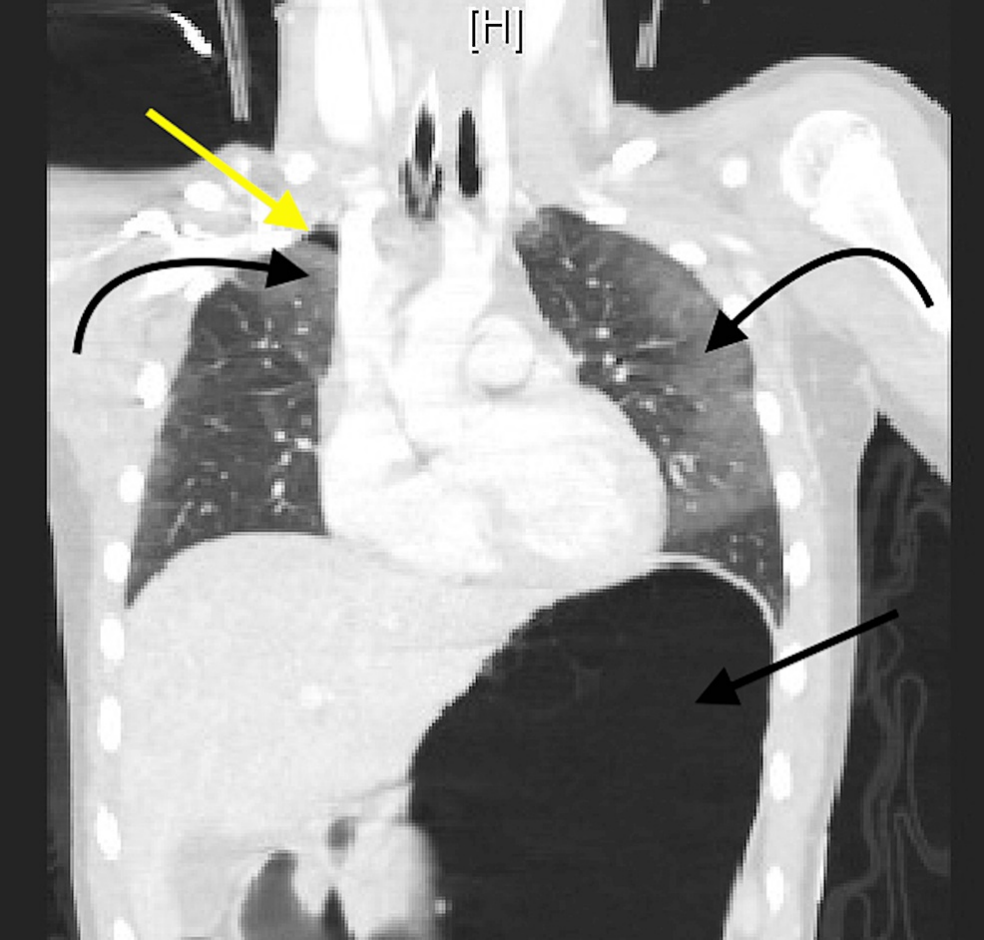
CT chest and abdomen showing right apical pneumatocele (yellow arrow), bilateral pulmonary contusions (curved black arrows), and large stomach (straight black arrow).
